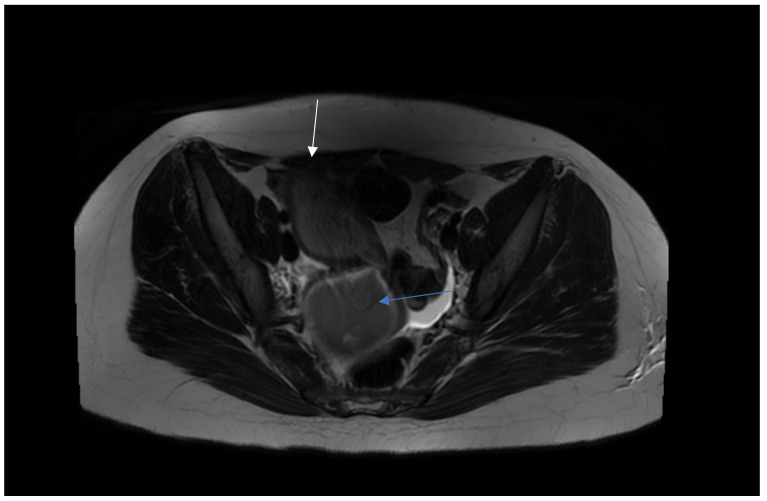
MRI presents hematocolpos (blue arrow) and hydrometrocolpos in right uterus (white arrow).
